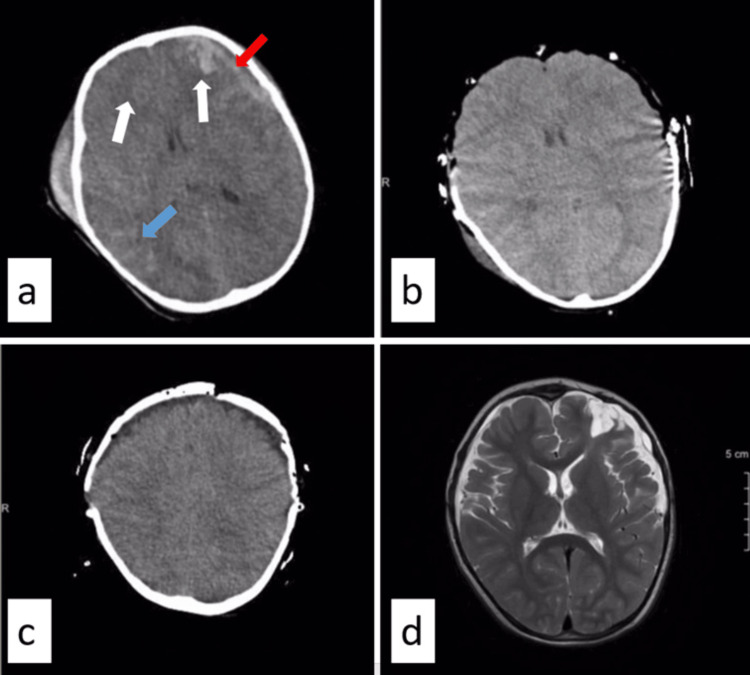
a. Computed tomography image at the time of admission, showing acute subdural haematoma in the left frontotemporal region (red arrow), contusion in the bilateral frontal region (white arrow), and traumatic subarachnoid haemorrhage in the right temporal region (blue arrow). b. Imaging after bilateral frontotemporoparietal decompression. c. Tomography image after cranioplasty with bones removed from the abdomen two months after trauma. d. T2-weighted magnetic resonance imaging six months after trauma. There is a significant subdural effusion on the left.
